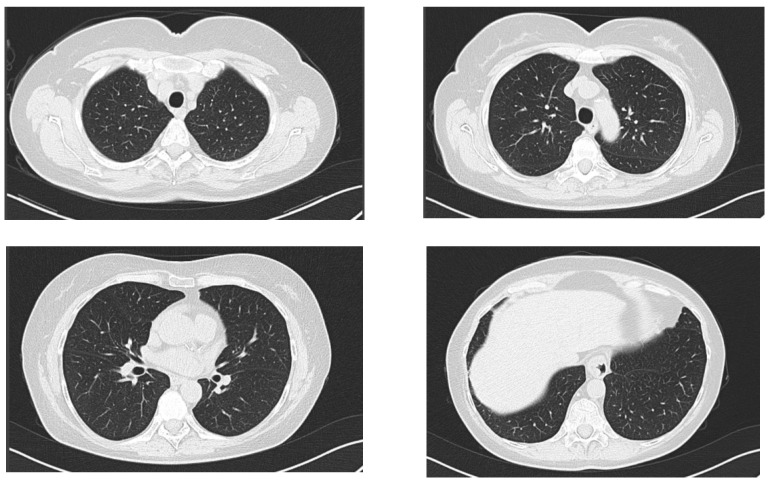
Axial computed chest CT of the second patient, lung window at the following levels: supraaortic trunks, aortic arch, pulmonary hila and base of the lungs, without any evidence of acute pulmonary lesions.
